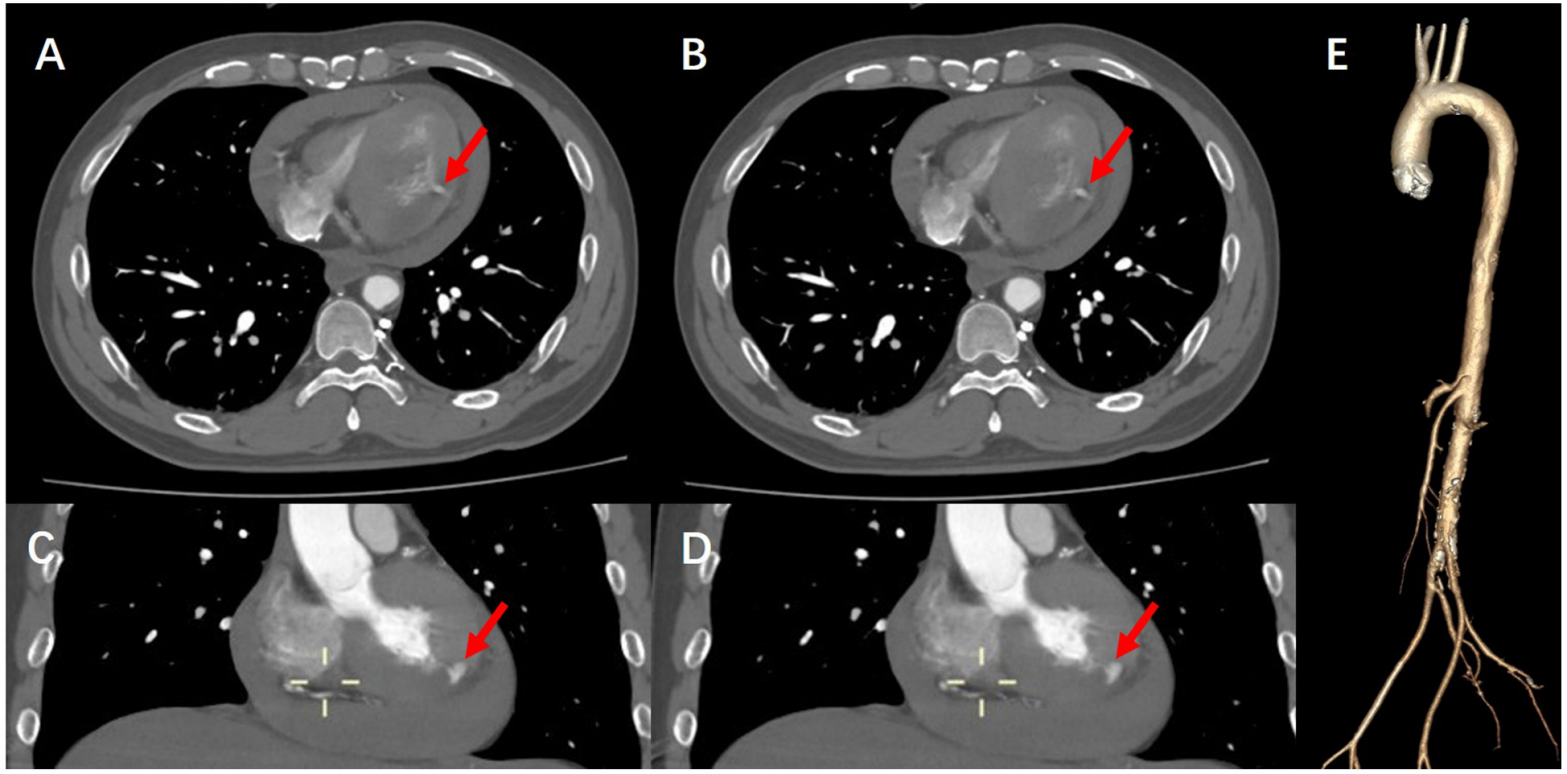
(A,B) CTA of the aorta demonstrate contrast extravasation into the myocardium, along with right-sided heart failure and pericardial effusion. (C,D) Coronal views of aortic CTA further reveal contrast leakage into the myocardial tissue. (E) Three-dimensional reconstruction of the aorta confirms the absence of aortic dissection, intramural hematoma, or other vascular anomalies.
